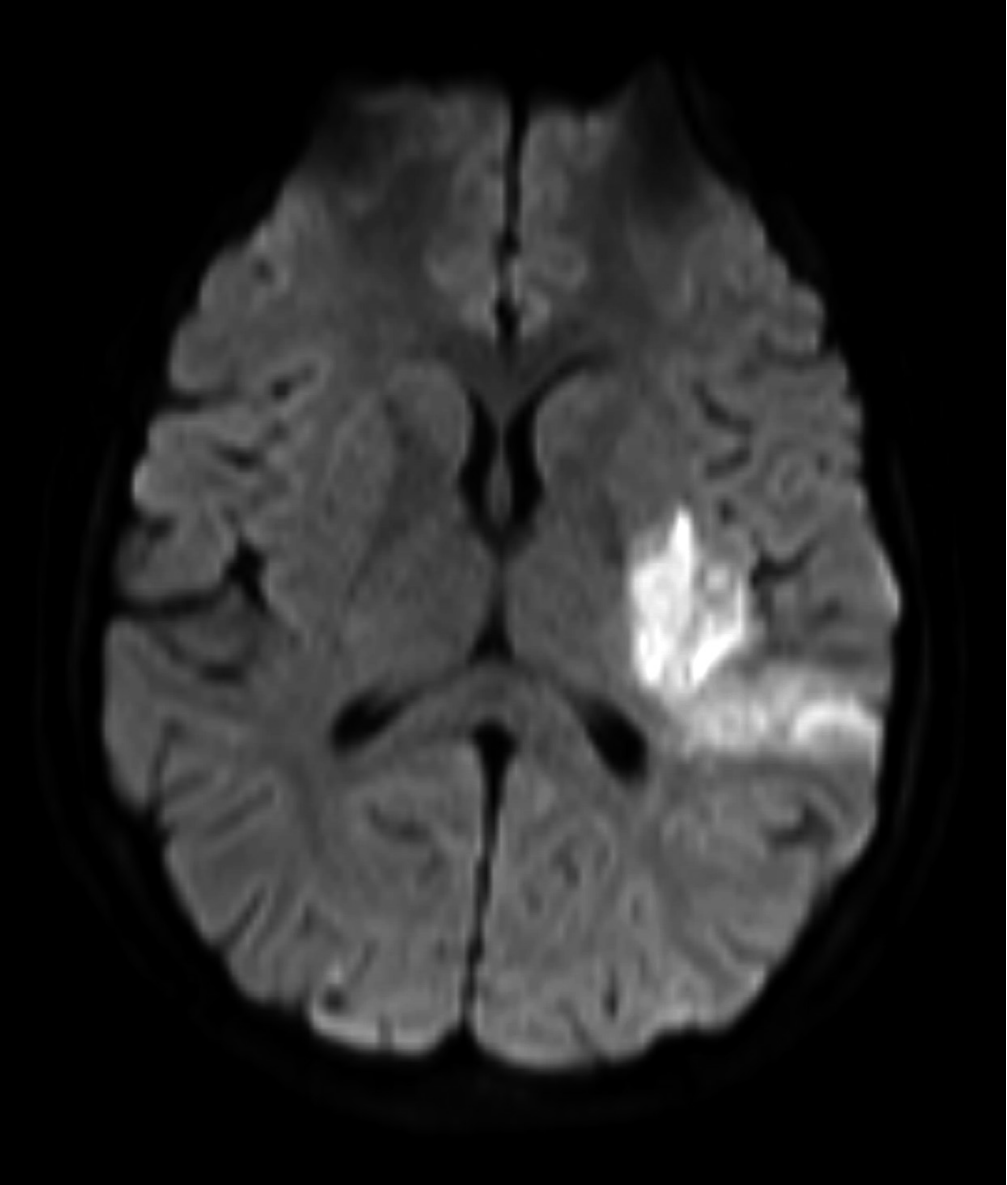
Dense Weight Imaging (DWI) sequence view showing infarction in left territory.
